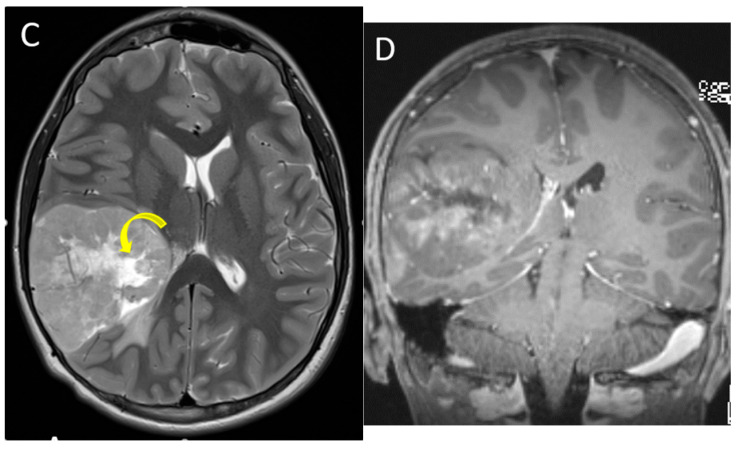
A 14-year-old male with H3 G34R mutant high-grade glioma. Axial DWI (A), axial SWI (B), axial T2 (C) and coronal post-contrast T1-weighted (D) images demonstrate a large mass in the right parieto-temporal lobes, with diffusion restriction of the solid portions, central areas of blood products and/or mineralization (arrow), isointense T2 signal with central necrosis (curved arrow), and heterogenous post-contrast enhancement.
